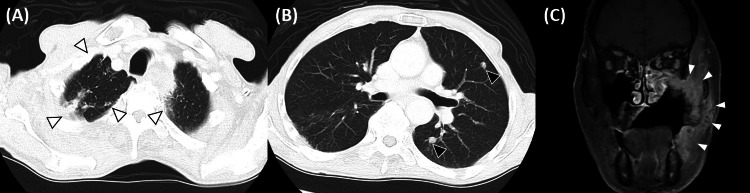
High-resolution CT and MRI images A, B) High-resolution CT of the chest after eight months of anti-tuberculosis (TB) treatment represented the decrease of opacities and old inflammatory change after TB (white and black arrowheads). C) Contrast-enhanced magnetic resonance imaging (MRI) of the head and neck region revealed the presence of an aggressive tumoral formation, extending from the left maxillary region to the masticatory muscle space, pterygopalatine fossa, suborbital fossa, accompanied by localized recurrence (white arrowheads).
